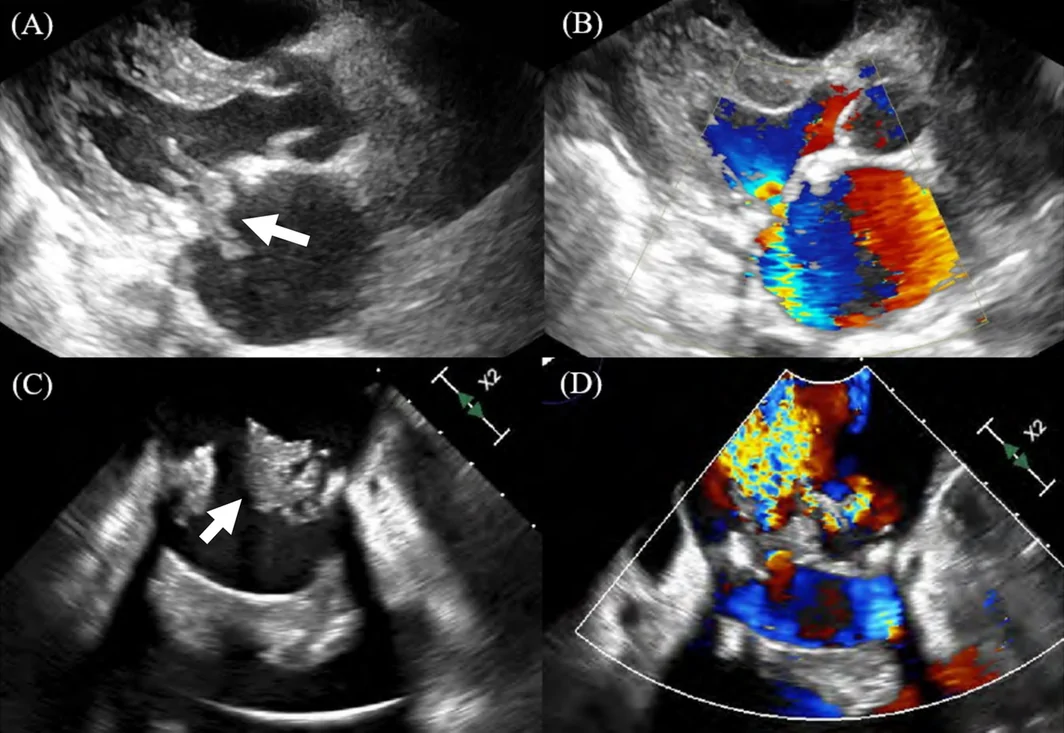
Transthoracic and transesophageal echocardiographic findings. (A, B) Transthoracic echocardiography demonstrating a mobile vegetation attached to the anterior leaflet of the mitral valve (arrow) with severe mitral regurgitation. (C, D) Transesophageal echocardiography showing generalized thickening of the mitral valve and a vegetation measuring > 10 mm in diameter (arrow).
